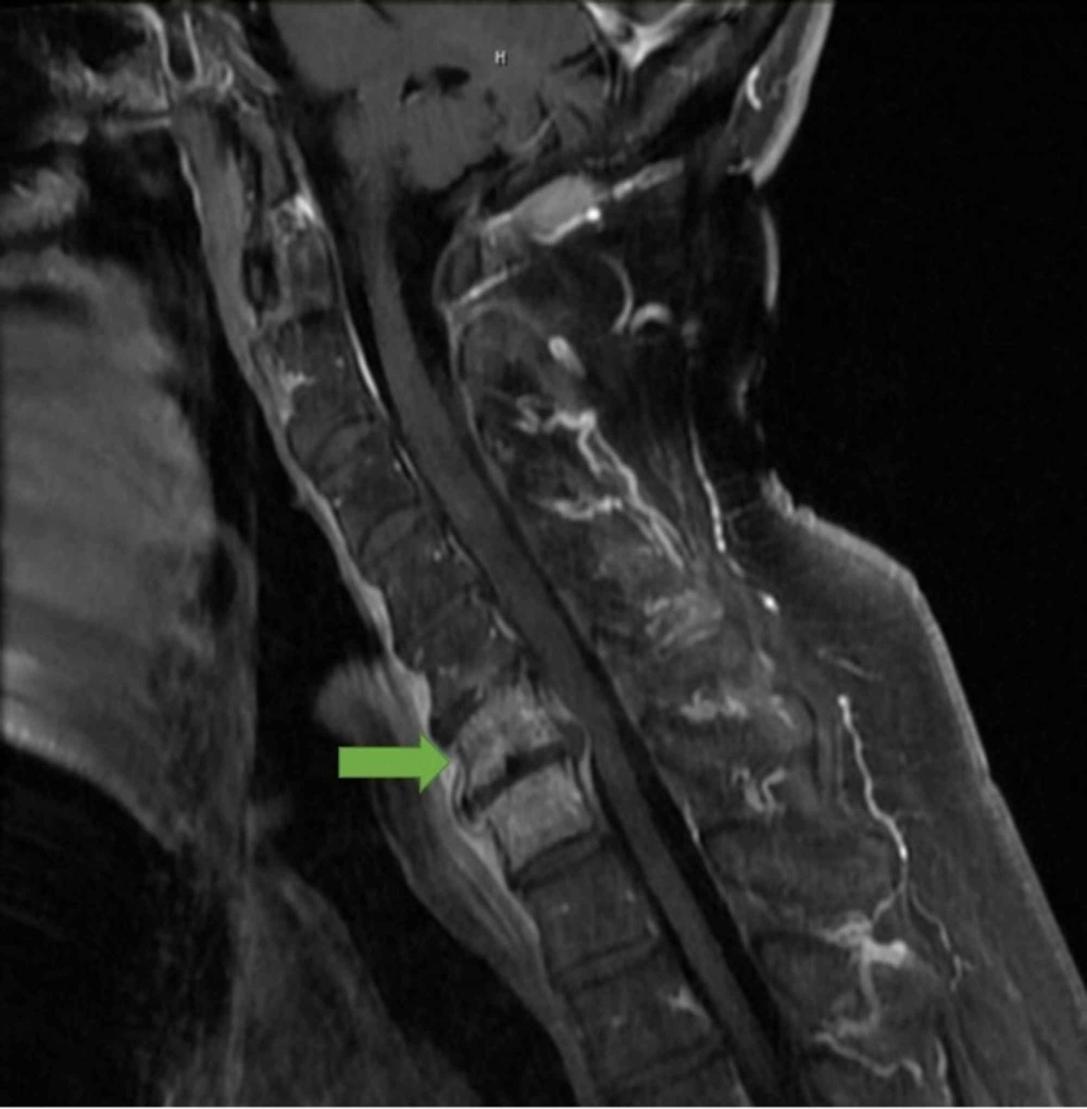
MRI of the spine with intravenous contrast showing cervical spine C6-C7 discitis/osteomyelitis
